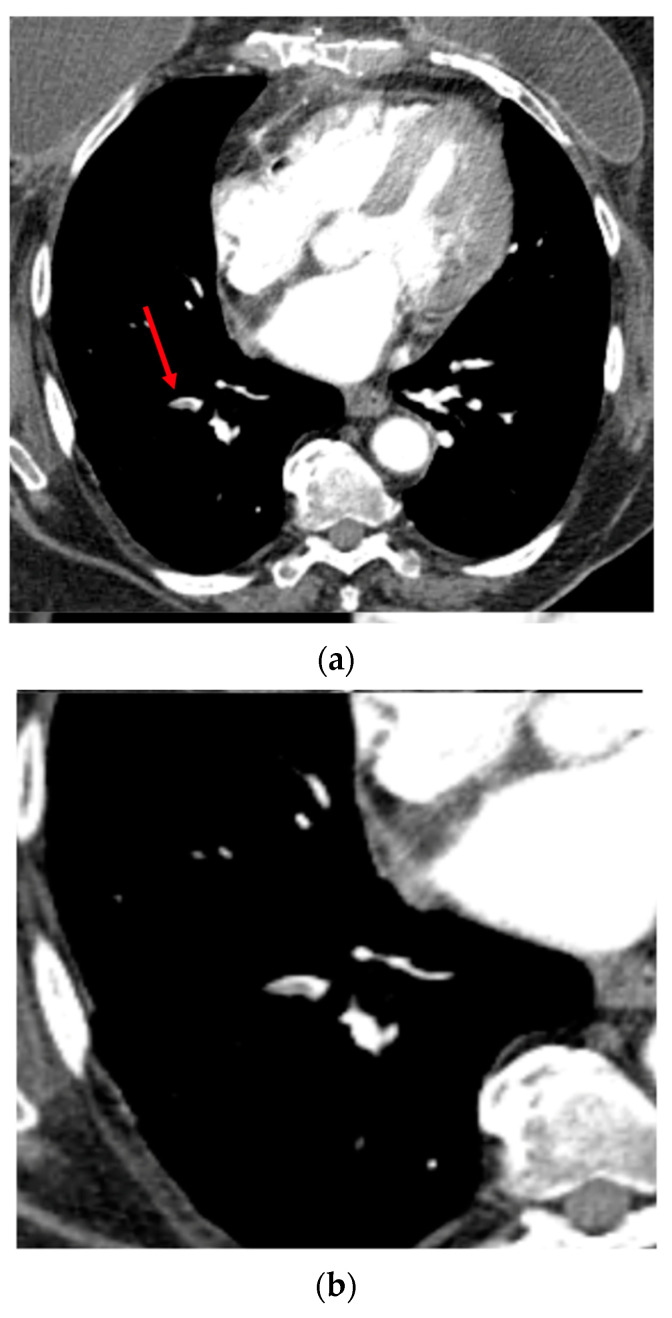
Axial CTA image. Example of segmental pulmonary embolus (PE) in the right lower lobe (filling defect within the arterial lumen), correctly detected by the CINA-PE software considered as a true positive case. Top (a): The red arrow shows the clot within the arterial lumen. Bottom (b): Axial image targeted on the right lower lobe.
